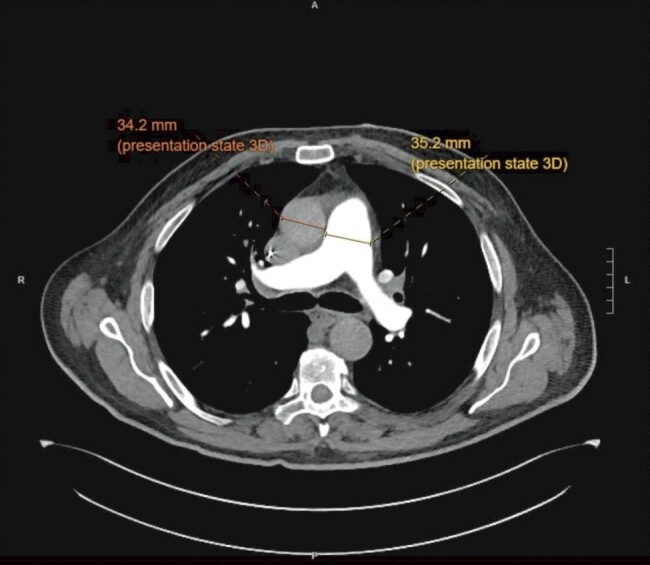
CT image showing the patient’s enlarged pulmonary artery (diameter 35.2 mm) compared with the aorta (diameter 34.2 mm).
